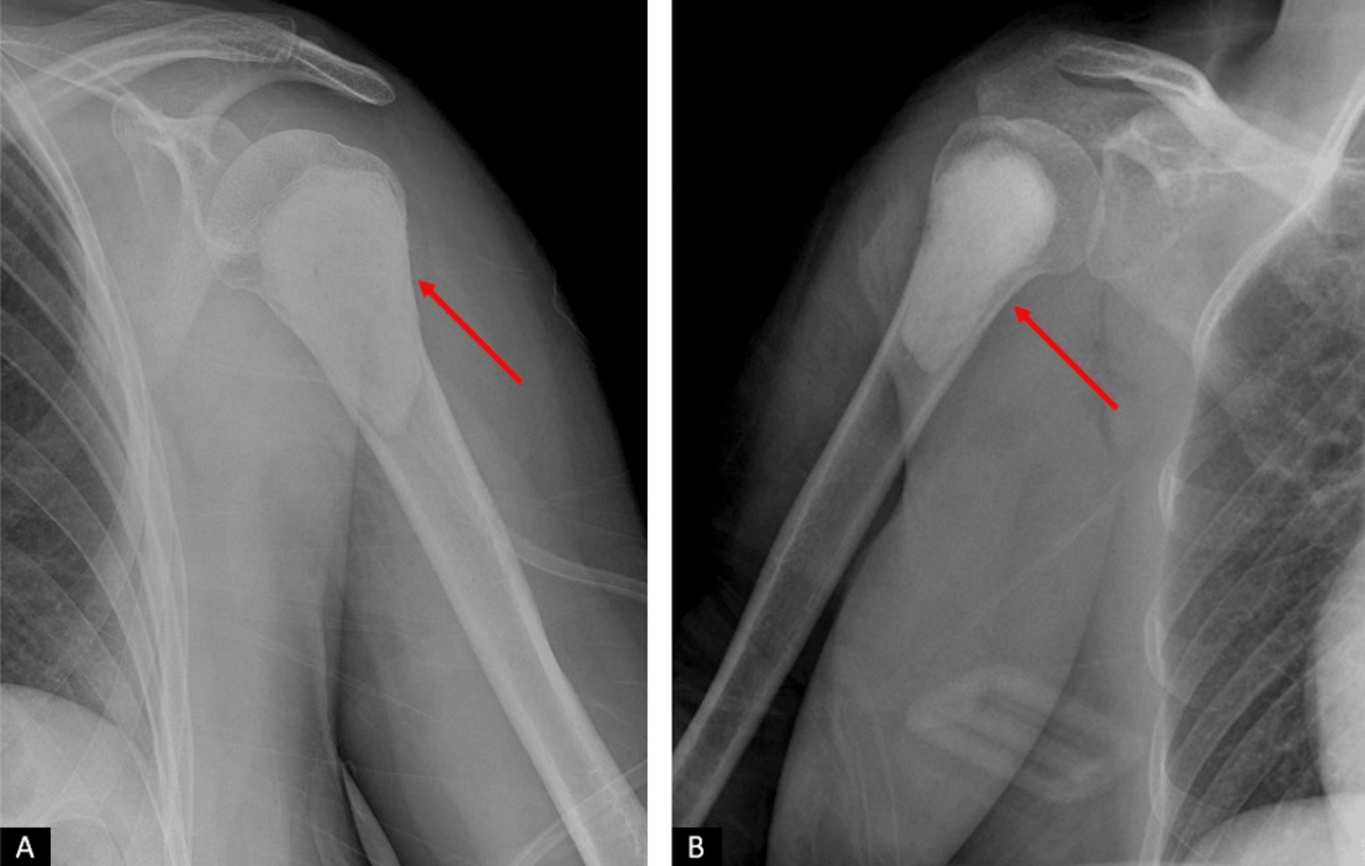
Postoperative radiographs of the patient. (A) Left shoulder AP view. (B) Right shoulder AP view. The bone regions where curettage and cementation were performed are indicated with red arrows.
